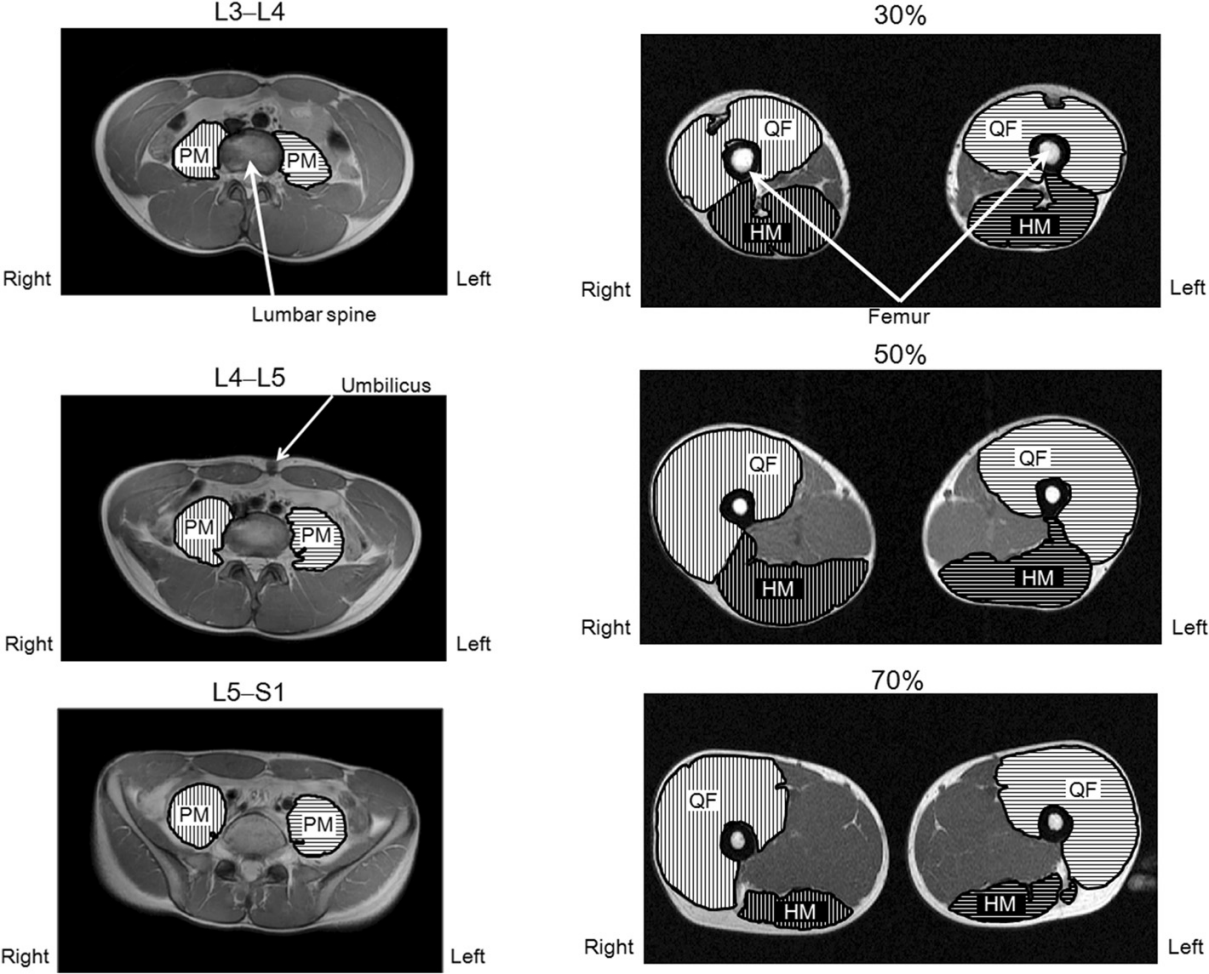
Typical cross-sectional magnetic resonance images of the psoas major muscle outlined at the L3–L4, L4–L5, and L5–S1 levels (L: lumbar spine, S: sacral spine), and those of the thigh muscles at the proximal 30, 50, and 70 % of the femur length
